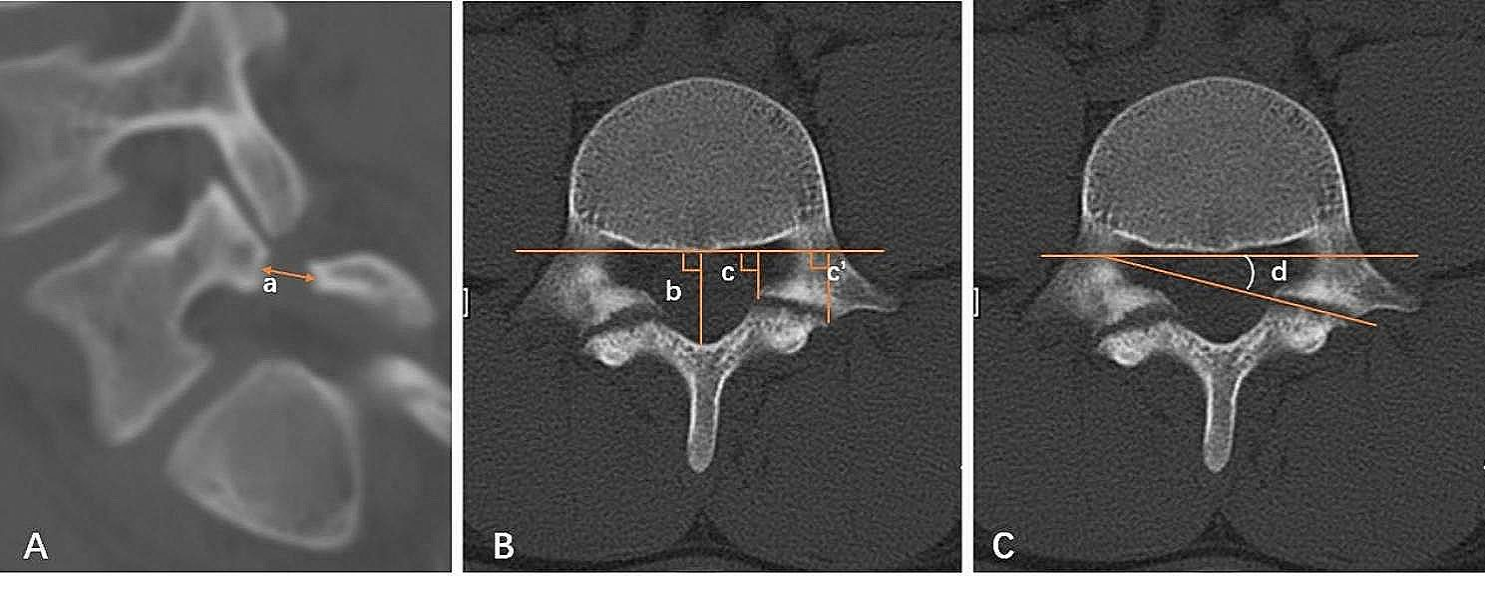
The width of the defect (segment a) is measured on reconstructed sagittal CT image in the middle of the pars interarticularis (A). The distance of the defect to the vertebral body is calculated on axial CT image by the following formula: distance = (c + c’)/2b (B). Angle of the defect (angle d) is the angle between the line of the posterior margin of the vertebral body and the line of the defect on the axial CT image (C)
